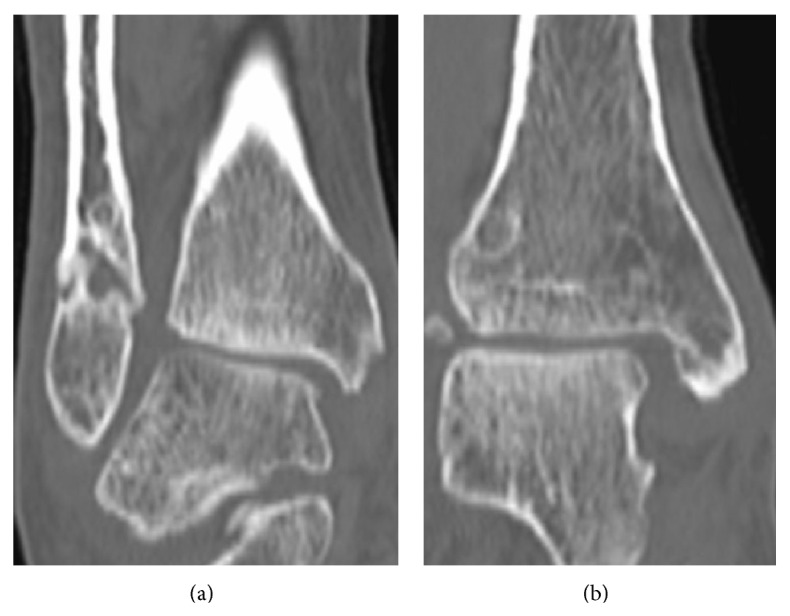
CT coronal view at the time of admission to our hospital. (a) Lateral malleolus was malunited, (b) in spite of medial malleolus being united.
